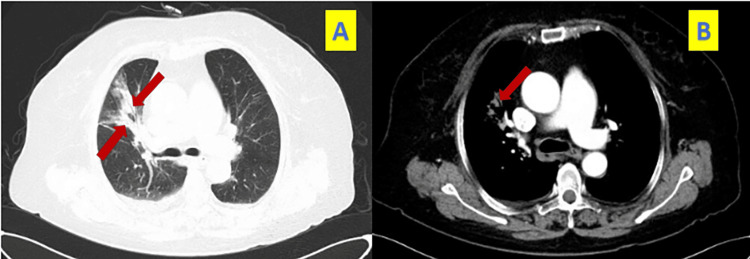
The patient's enhanced chest CT revealed an enlargement in the front-to-back diameter of the thorax, along with various patches and consolidation in the middle lobe of the right lung. Additionally, a positive air bronchogram was observed, highlighted by the red arrow in images A and B corresponding to the CT lung and mediastinal windows, respectively.
